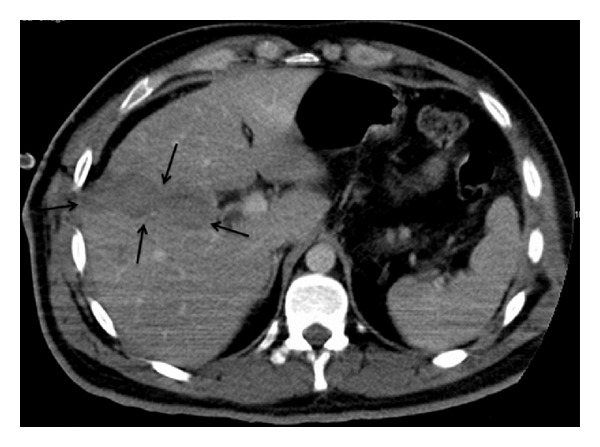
CT imagination of liver rupture.
